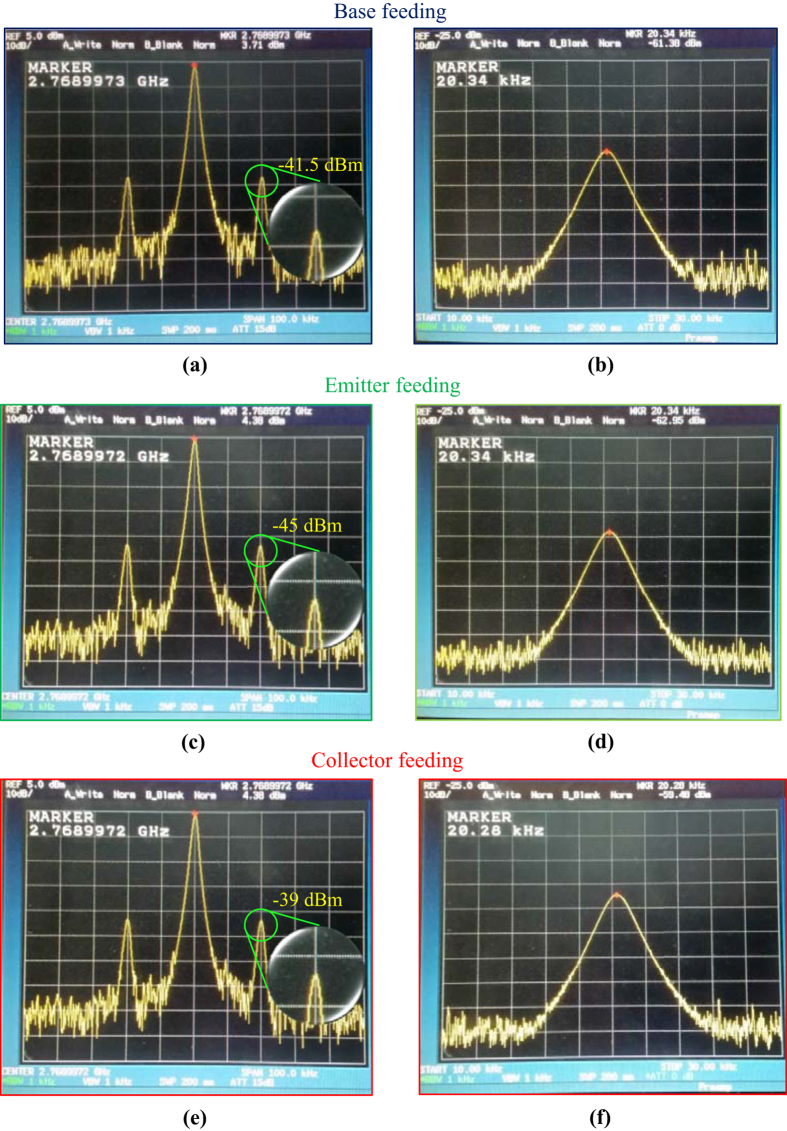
Output spectrum of the modified Colpitts oscillator. Output spectrum in a natural frequency band and a Doppler frequency band when feeding Doppler-shifted signal into the base (the spectra are shown in (a,b)), the emitter (the spectra are shown in (c,d)), and the collector (the spectra are shown in (e,f)) of the transistor.
